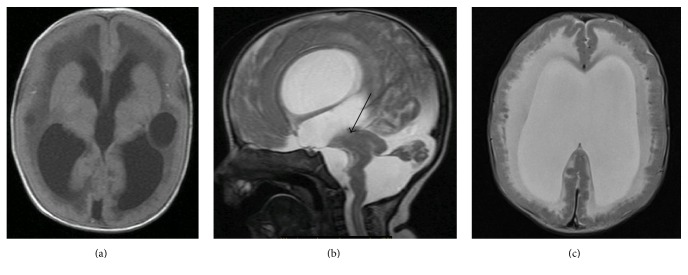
(a) Axial FLAIR: agyria enlarged ventricle, bilateral periventricle cystic hypodenisty, and delayed myelination of cerebral cortex. (b) Sagittal T2: enlarged lateral and third ventricle. Hypoplastic cerebellum, large posterior fossa CSF fluid space. There is no visible aqueduct (arrow), kinked pontomesencephalic kink. (c) Axial T2-weighted image reveals typical changes related to cobblestone lissencephaly. White matter is diffusely hyperintensity. Enlarged ventricle.
